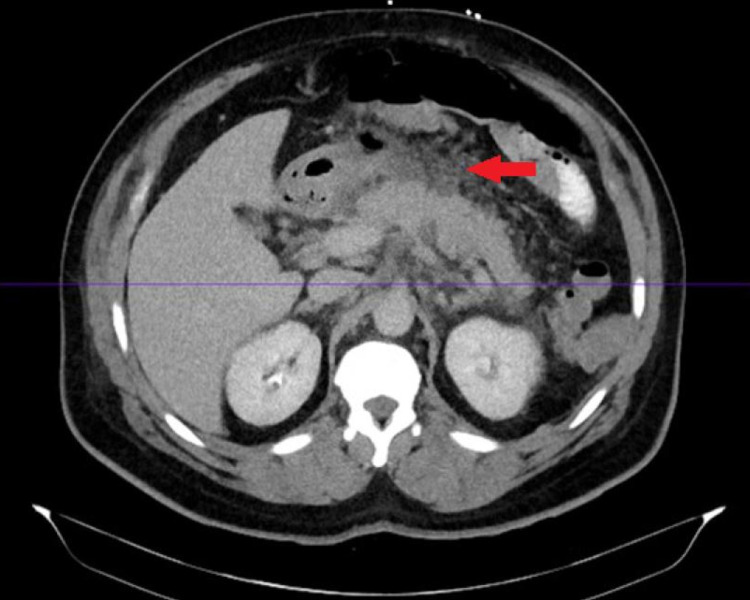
Computed tomography of the abdomen with intravenous contrast. Red arrow showing increased peripancreatic fat stranding, fluid, and possible early phlegmon suggestive of acute pancreatitis. An organized abscess or other fluid collection is not seen.
